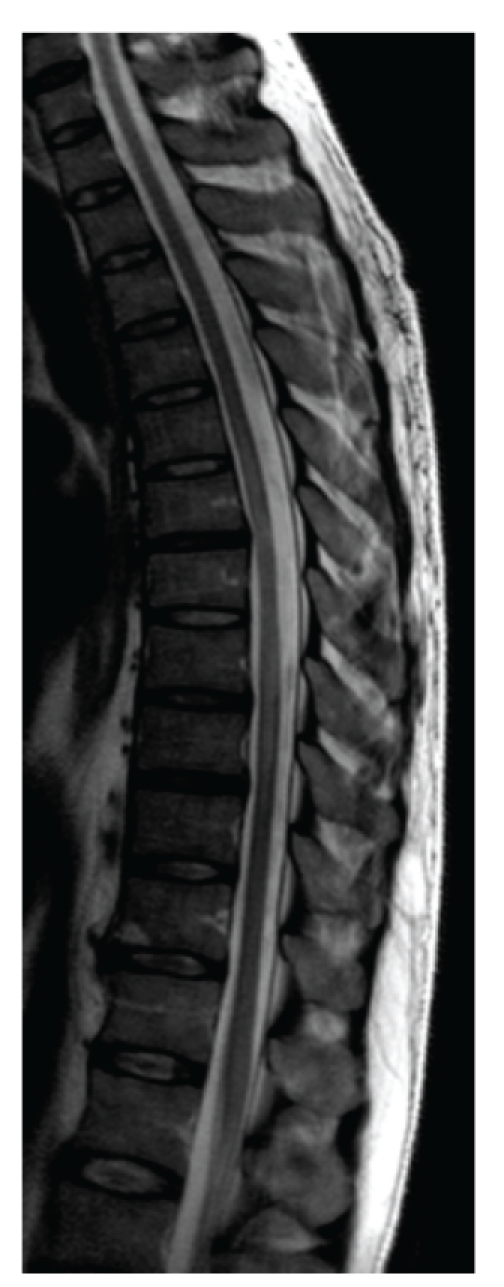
Sagittal T2-weighted spinal MRI 2 months later shows complete resolution of the lesion.
